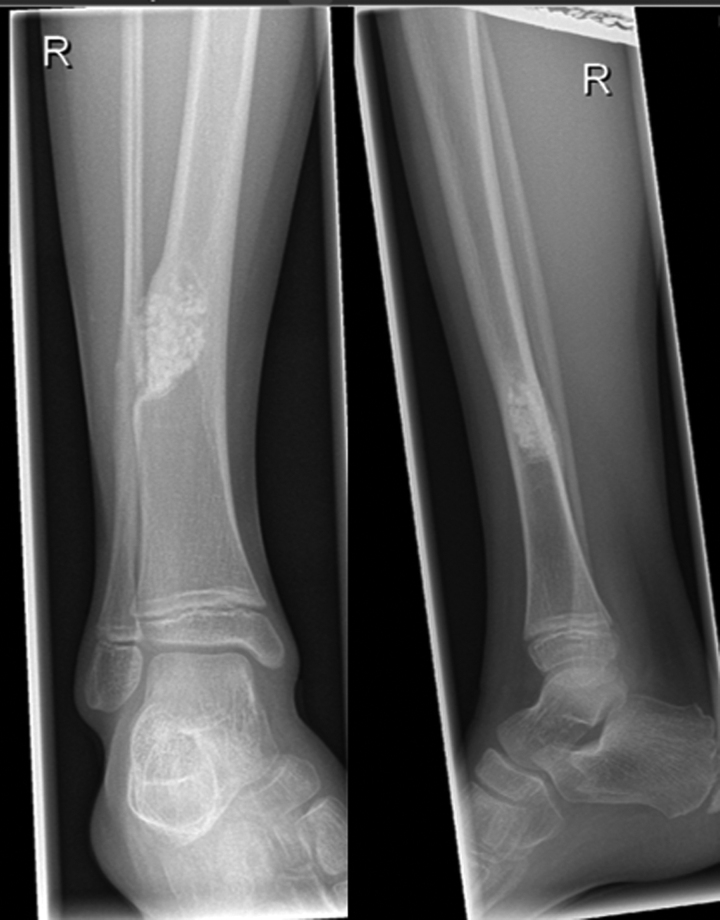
Radiographic image after removal of the cement plombage and filling with osteoconductive material at latest follow-up.
